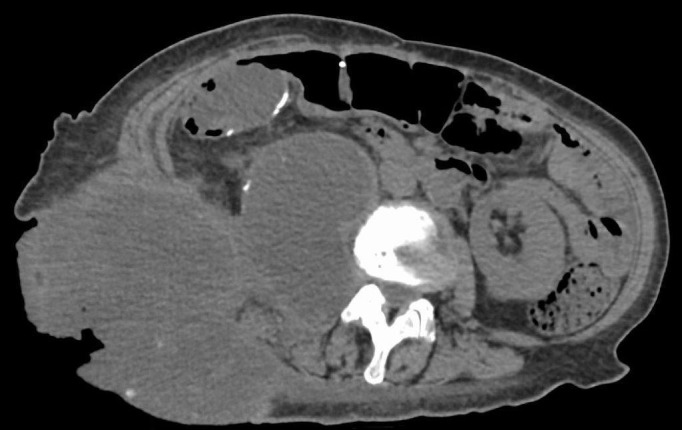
Unenhanced axial CT image showing confluent intra- and extra-abdominal disease
